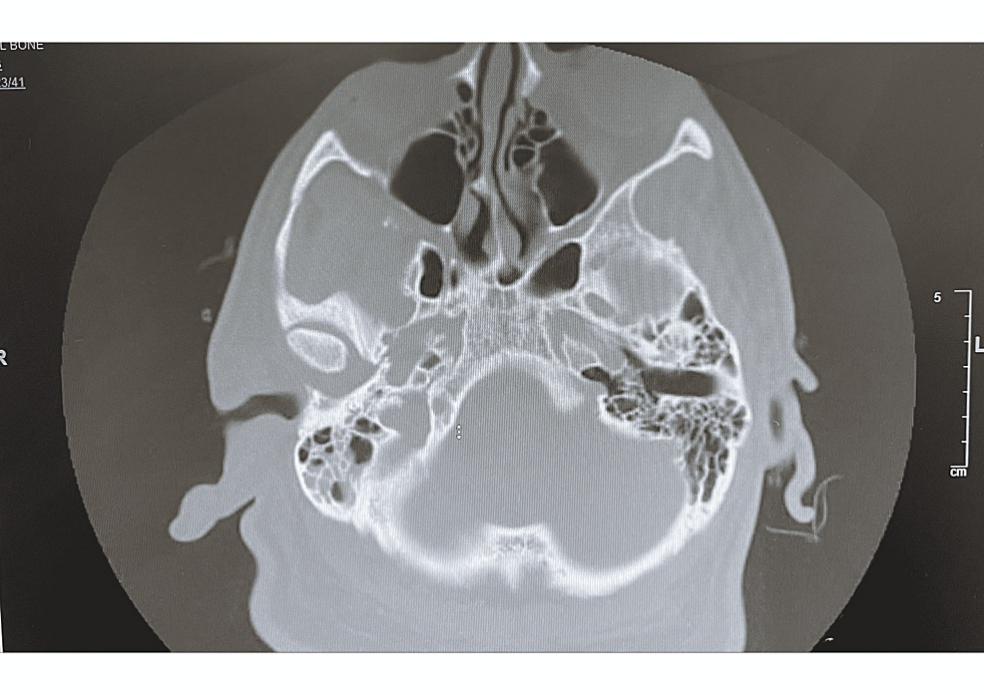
CT ears without contrast showed fluid-filled inferior right mastoid air cells to support the case presentation.
